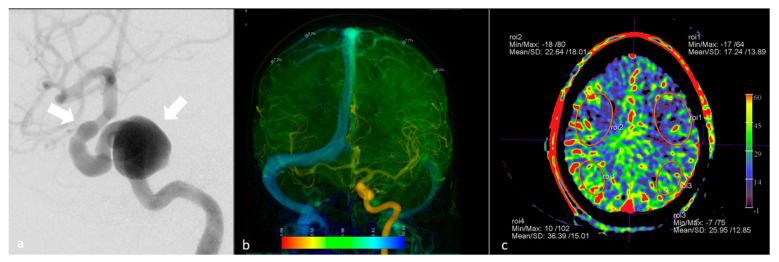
FD CT PBV imaging during test occlusion in a 57-year-old female with internal carotid artery aneurysms. (a) Depiction of the aneurysms (white arrows) on a lateral view DSA image. (b) Color-coded DSA image in anteroposterior view during test occlusion shows almost synchronous filling of the cortical veins, indicating the patient’s potential tolerance to PVO. (c) PBV MIP reformations can be utilized for plotting multiple ROIs in both hemispheres in a standardized fashion.
